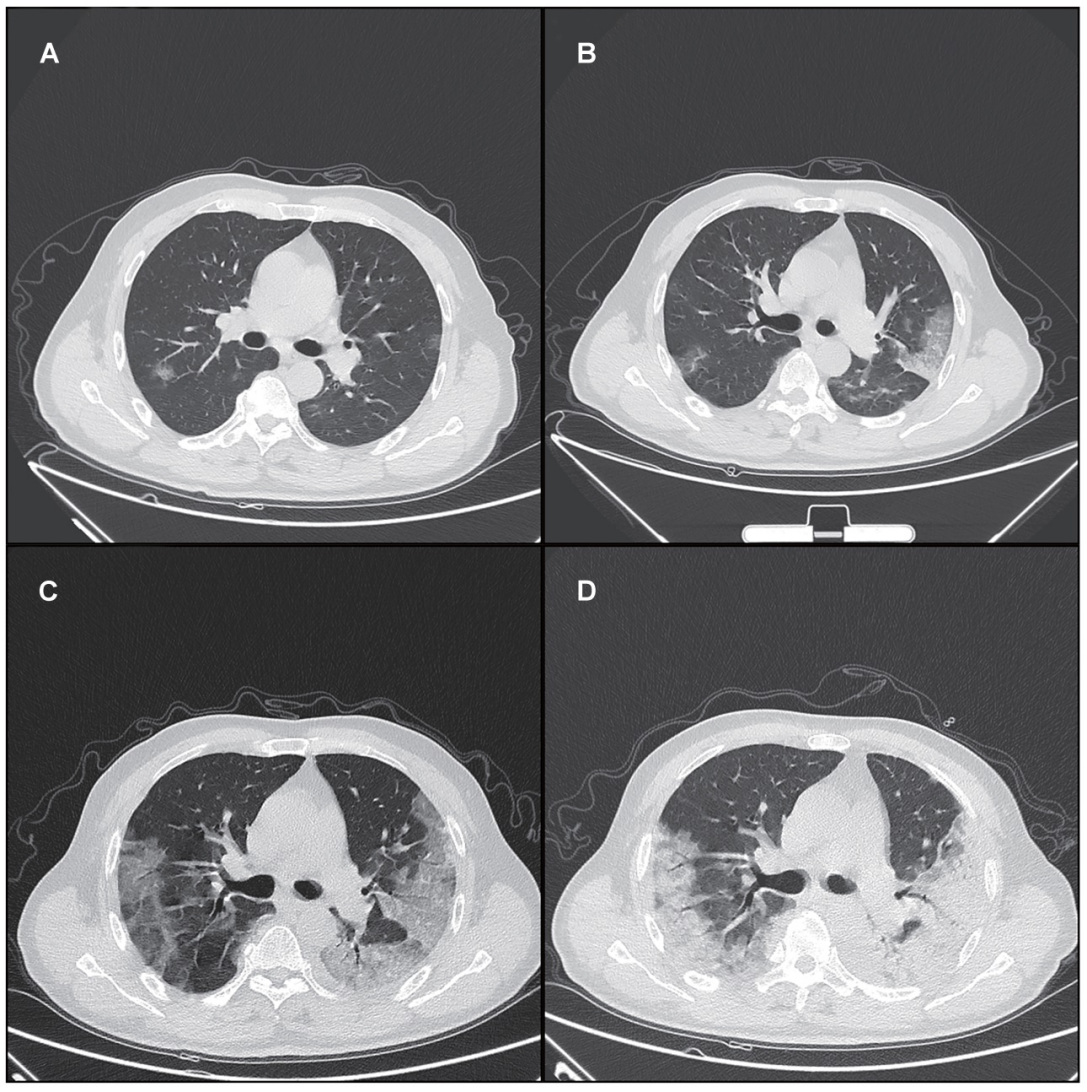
Typical series of chest CT demonstrations in a 65-year-old male patient with COVID-19 in different periods. (A) In the first period (0 - 5 days), GGO; (B) In the second period (6 - 10 days), a crazy-paving pattern (GGO with superimposed inter- and intralobular septal thickening); (C) In the third period (11 - 15 days), a mixed pattern (GGO, crazy-paving pattern and consolidation); (D) In the fourth period (16 - 20 days), a mixed pattern (crazy-paving pattern and consolidation). All images have the same window level of - 600 and window width of 1600. GGO: ground glass opacity.
